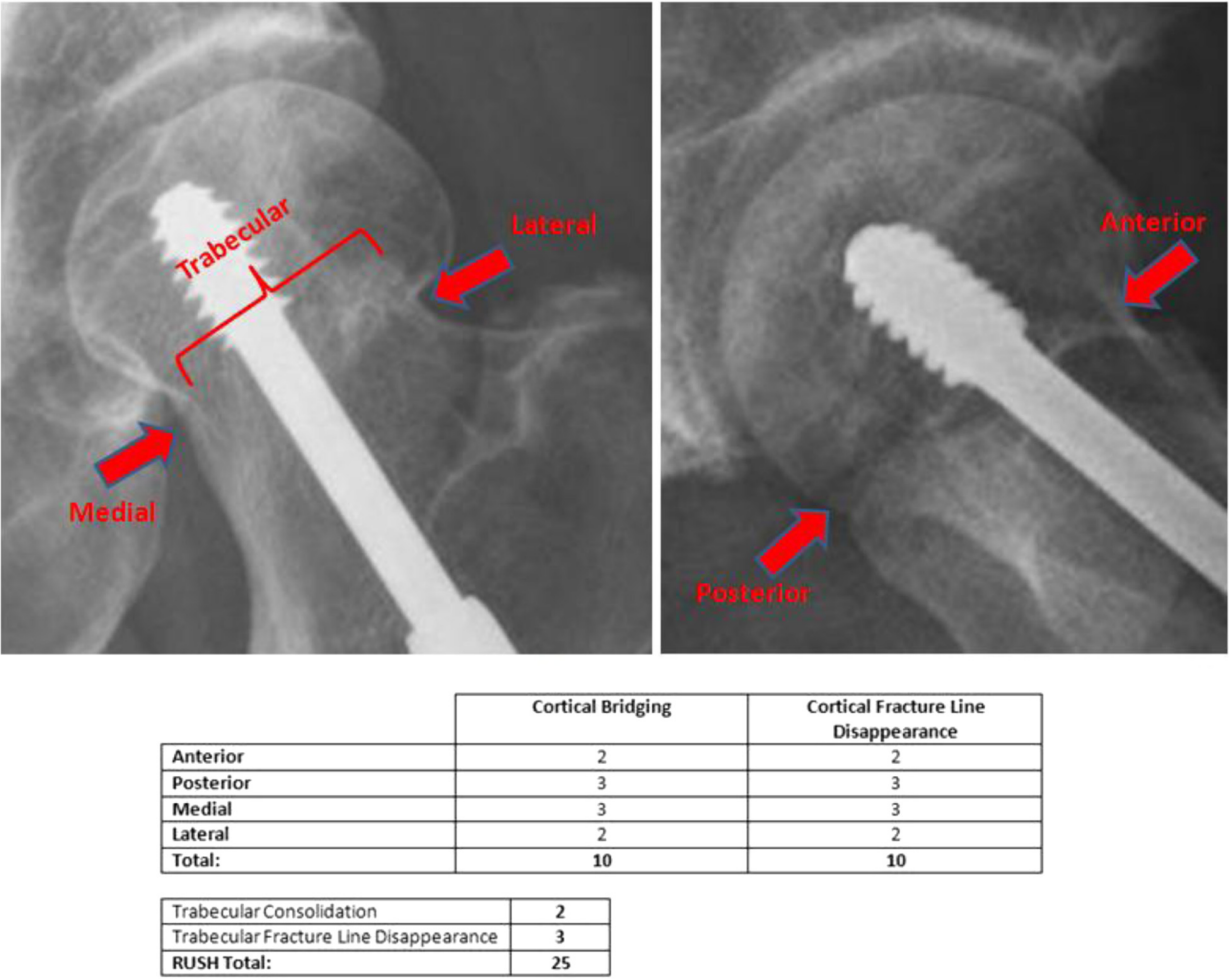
Late post-operative radiographs and RUSH assessment.
